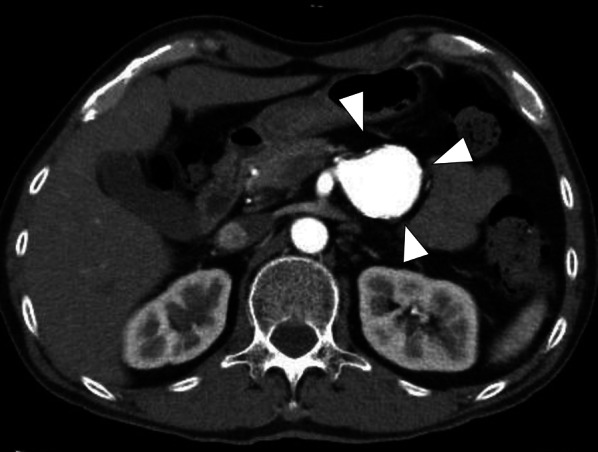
Arterial-phase computed tomography scan showing a homogeneously contrasted aneurysm (white triangle)
